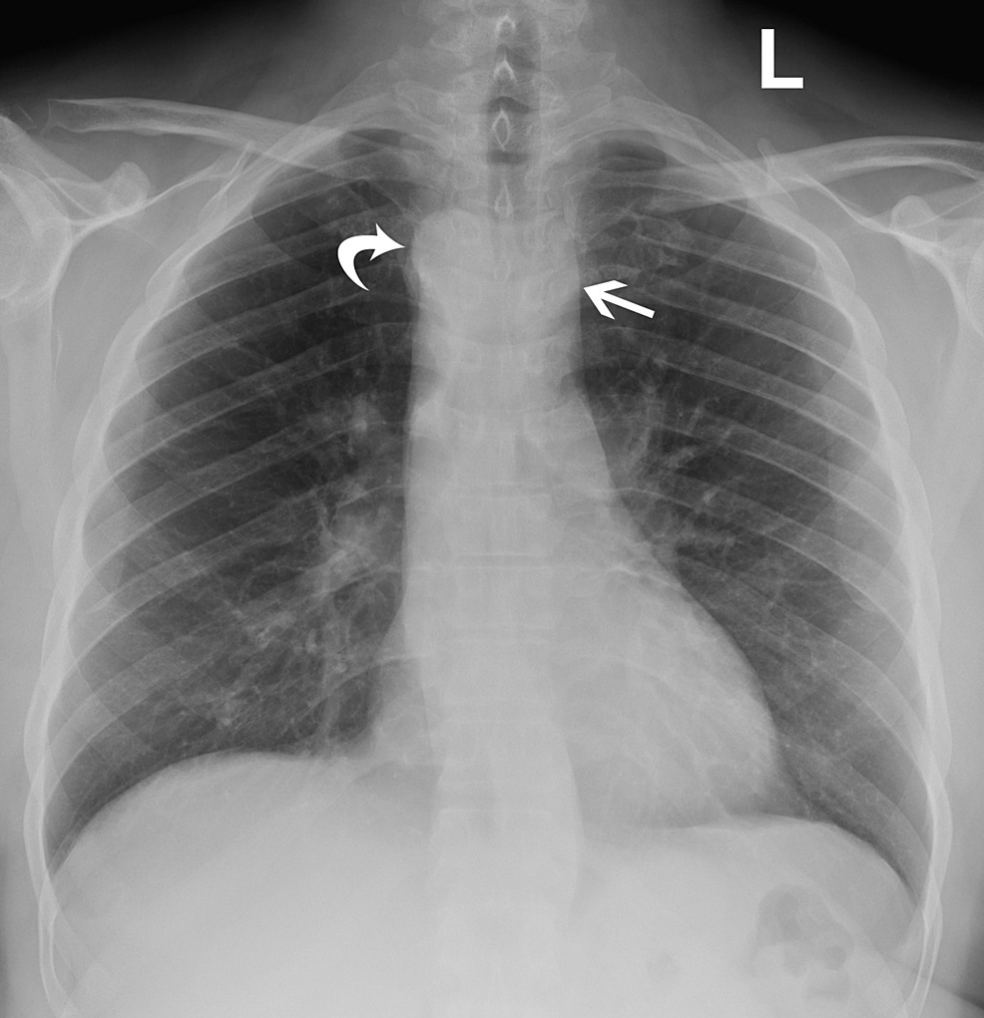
Posteroanterior chest radiograph shows right (curved arrow) and left (straight arrow) paratracheal opacities representing a double aortic arch.
